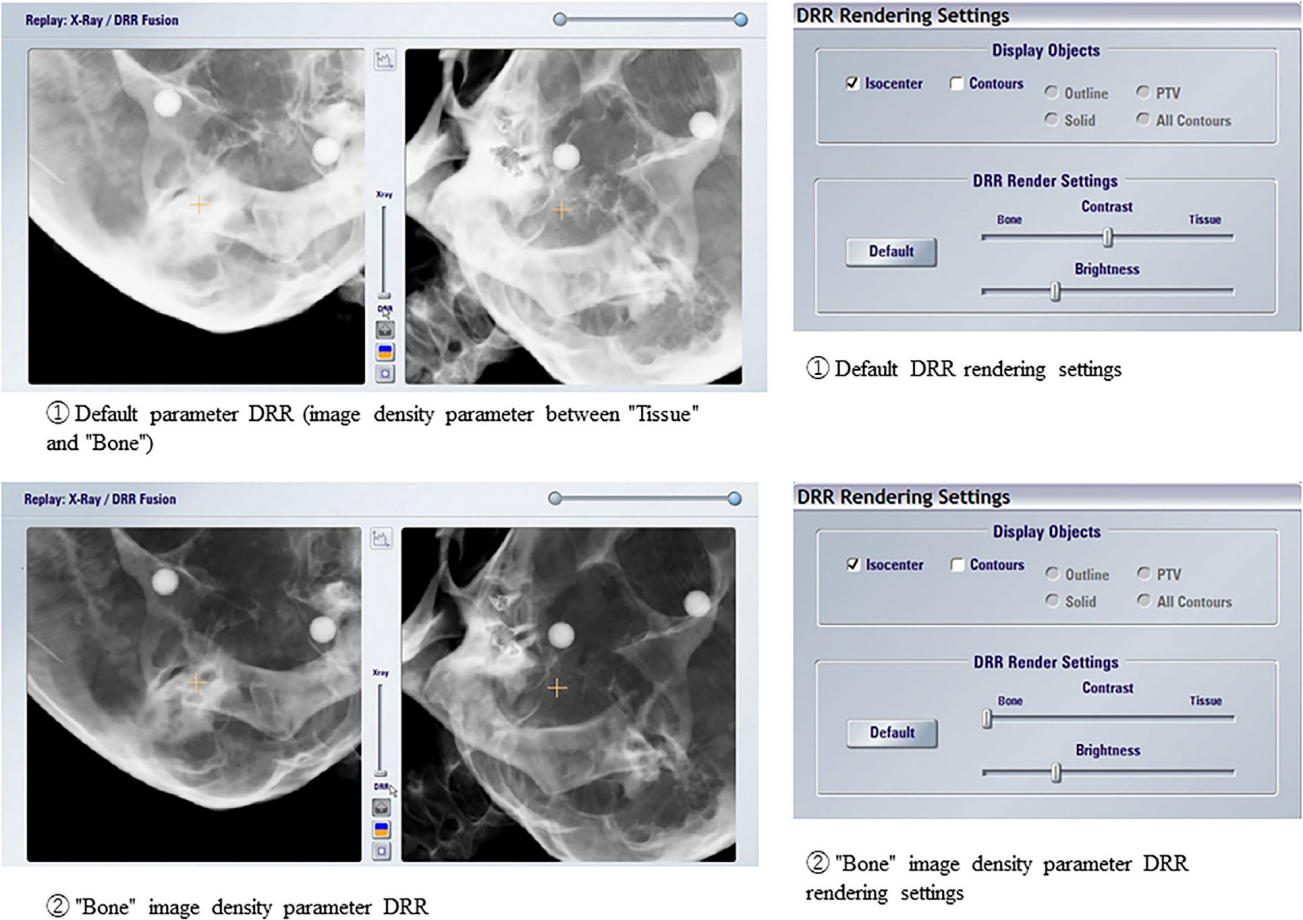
An example of two types of image density adjustment parameters. ① Default parameter digitally reconstructed radiograph (DRR) (image density parameter between “tissue” and “bone”). ② “Bone” image density parameter DRR
